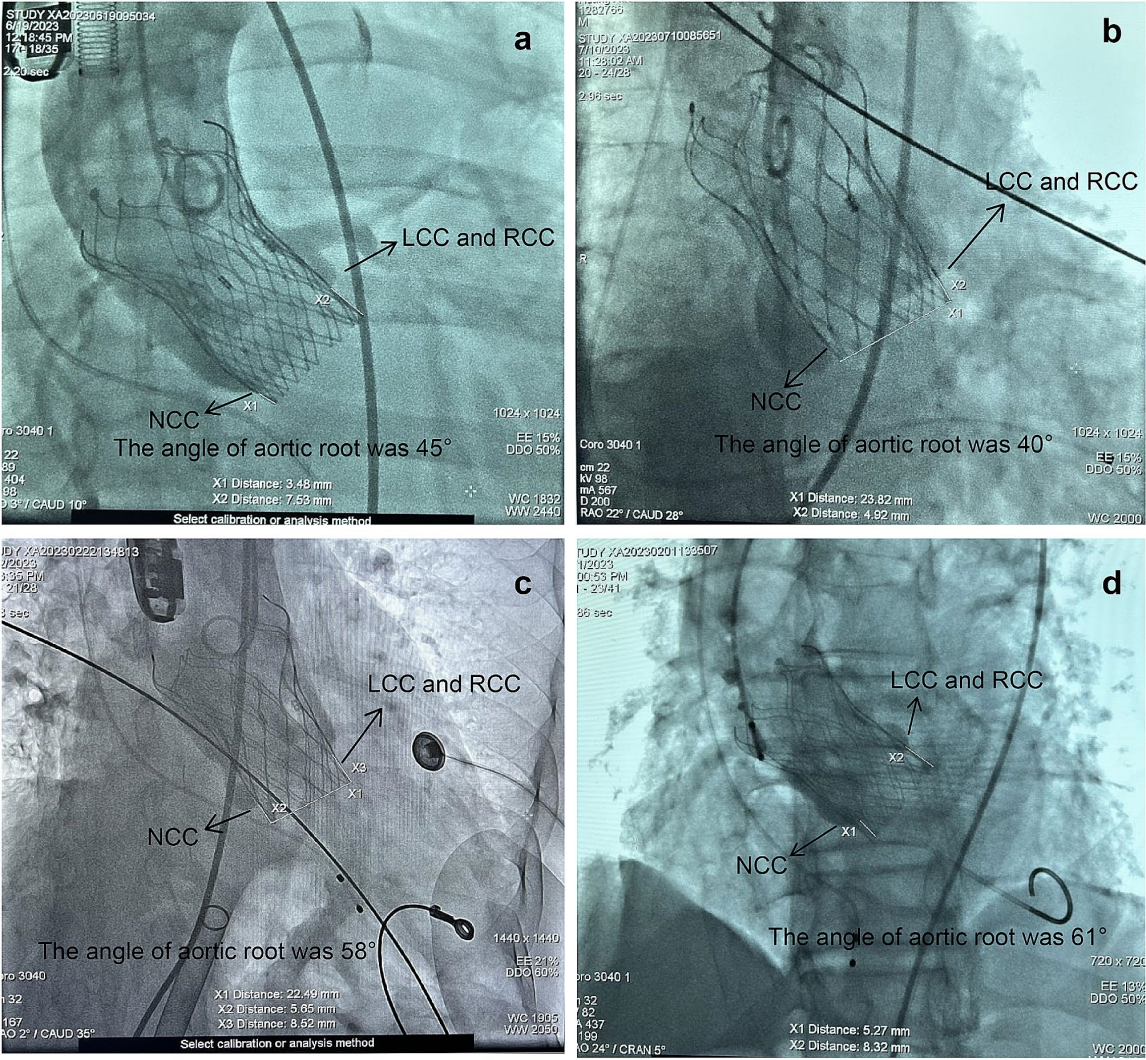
Valve implantation depth This depth is defined as the distance from the lower edge of the valve stent device after its release to the lowest point of the NCC, which is mostly consistent with the data measured by TEE (a) Valve implantation depth is 3.48 mm without new-onset heart block; (b) Valve implantation depth is 0.00 mm without new-onset heart block; (c) Valve implantation depth is 5.65 mm with new-onset heart block and need for PPI; (d) Valve implantation depth is 5.27 mm with new-onset heart block and need for PPI LCC: left coronary cusp; NCC: non-coronary cusp; RCC: right coronary cusp; TEE: transesophageal echocardiography
